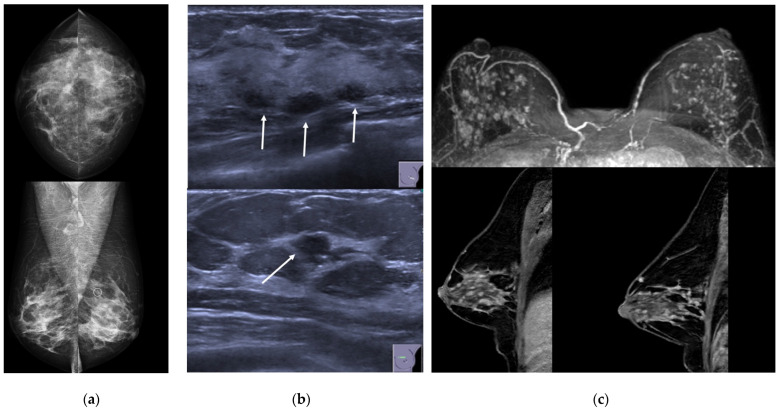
A 43-year-old woman presenting with unilateral bloody nipple discharge. (a) Mammography in cranio-caudal and medio-lateral views did not show any mass or asymmetric density, but only a millimetric cluster on calcification in the upper-outer quadrant of the left breast (circle). (b) Ultrasound demonstrated multiple small masses with ductal relation (arrows). (c) MRI showed multiple enhancing masses associated to ductal contrast enhancement with a “string of pearls” appearance.
